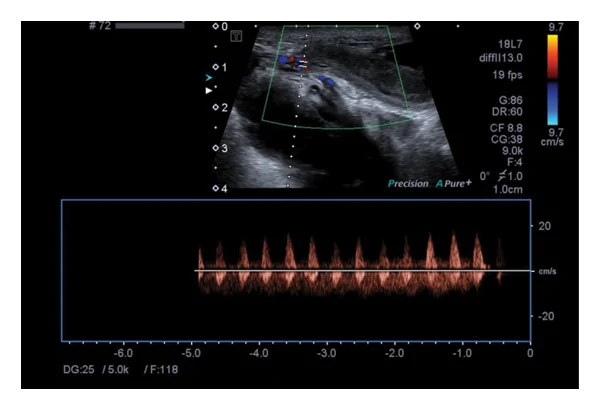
Color Doppler and flow waves proximal to interruption site.
